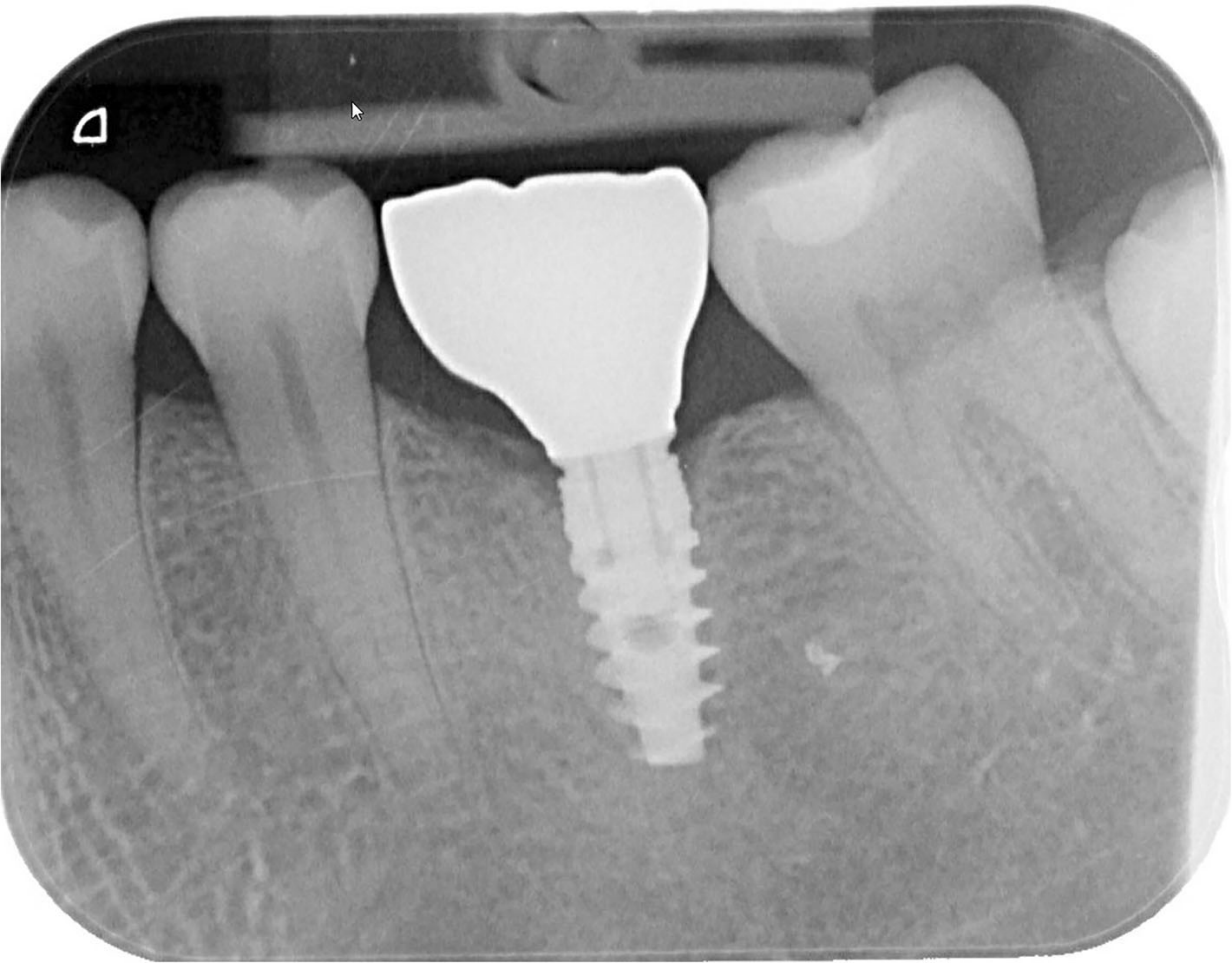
Intraoral radiograph of an immediately placed post-extractive dental implant with a full contour zirconia restoration after 1 year (same patient as in Fig. 1)
